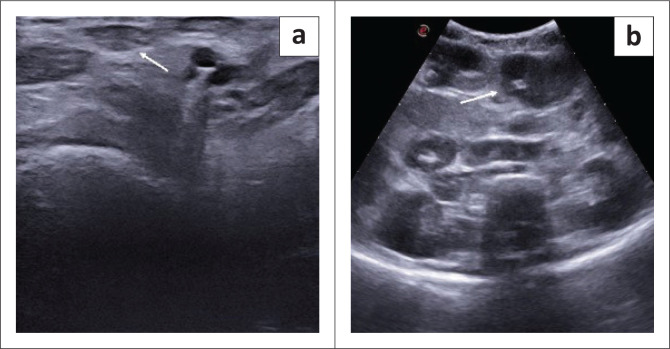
Ultrasound of the groin (a) and abdomen (b) in an 8-year-old male with disseminated tuberculosis showed (a) enlarged inguinal nodes (arrow) and (b) multiple enlarged abdominal lymph nodes (arrow).
